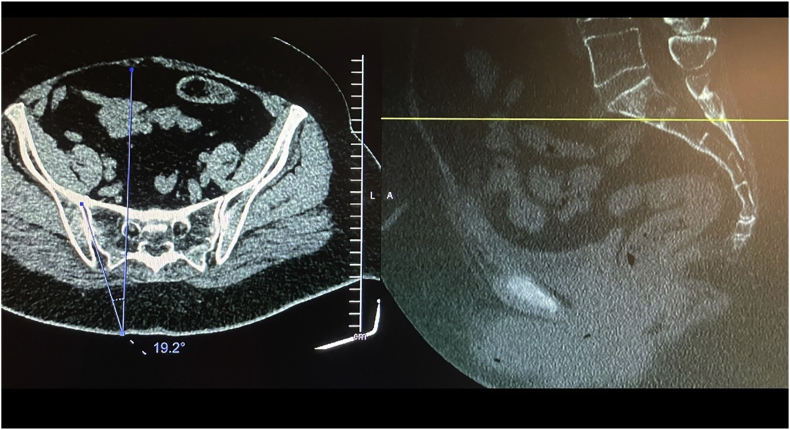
Contralateral oblique angle measurement. This figure depicts how the contralateral oblique angle was measured on CT pre-operatively.
